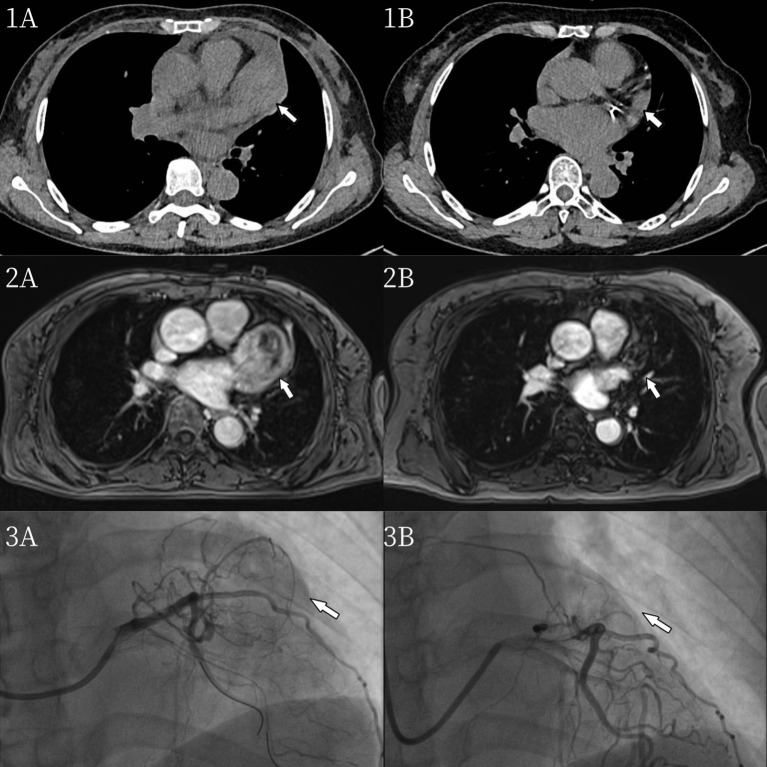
(A) Chest CT revealed a soft tissue tumor of approximately 50×40×30 mm in the pericardial region superior to the left ventricle. (B) One year after interventional embolization, the pericardial tumor significantly decreased in the Chest CT. (A) Cardiac MRI confirmed a pericardial tumor in the basal left ventricular region. (B) One year after interventional embolization, the pericardial tumor significantly decreased in the Cardiac MRI. (A) Coronary angiography confirmed a fistulous tract from the circumflex artery supplying the pericardial tumor. (B) Coronary angiography confirmed the pericardial tumor significantly decreased.
